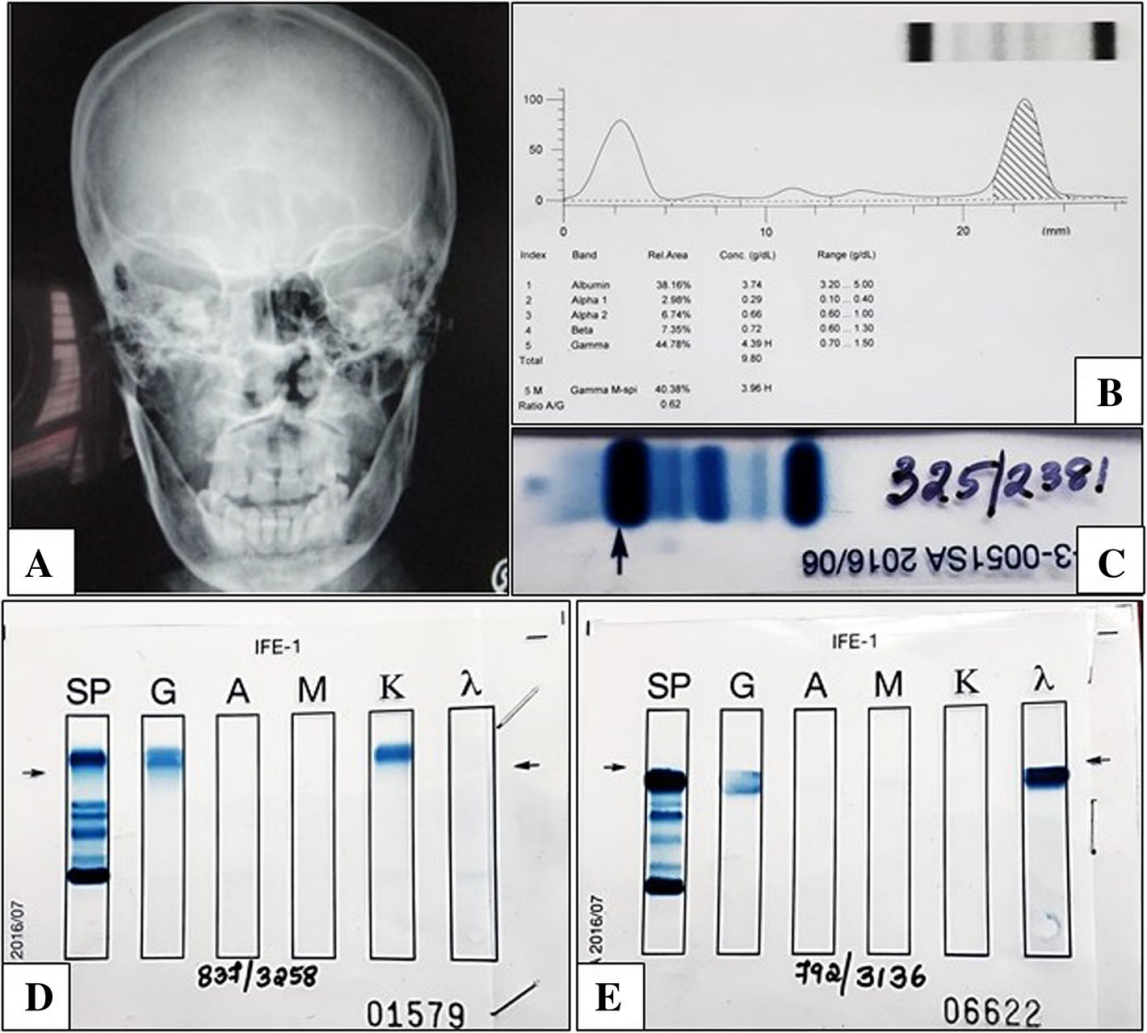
a Radiograph of plasmacytoma of right paranasal sinus presenting as a destructive osteolytic lesion (PA view) b & c: Serum protein electrophoresis with a significant M band suggecting paraproteinemia. d A case of SP with monoclonal kappa detected on IFE. e A case of SP with monoclonal lambda detected on IFE
